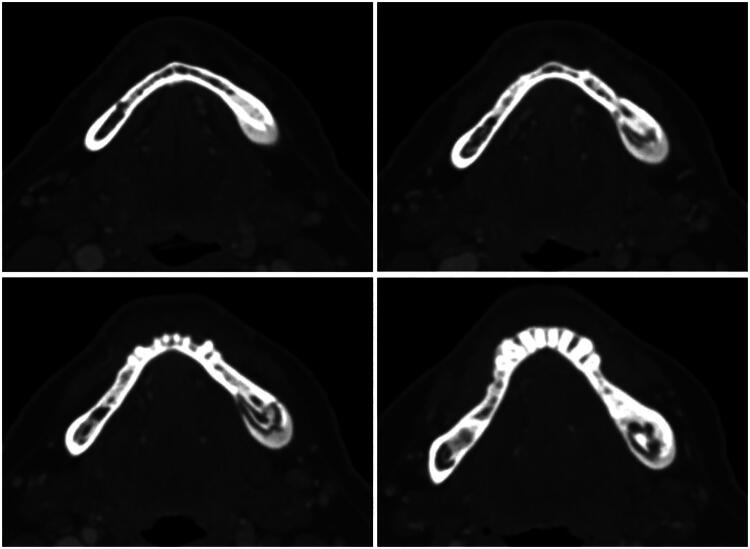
Computed tomography (CT) of the mandible demonstrating an osseous lesion involving the left paramedian body with features suspicious for chronic osteomyelitis. Note the partially extruded bony sequestrum and cortical irregularity, consistent with medication-related osteonecrosis of the jaw (MRONJ).
